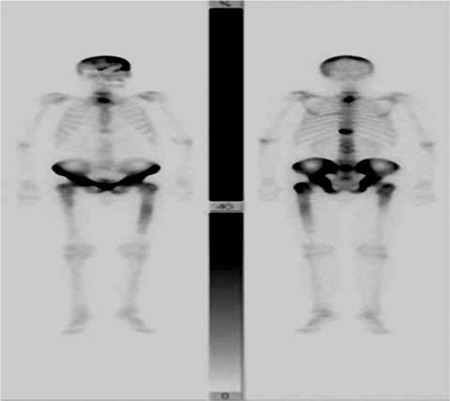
Dense and diffuse increased osteoblastic activity in the cranium; all pelvic and sacroiliac joints; the C7, T1-2, and T11 vertebrae of the vertebral column; and both proximal femurs on technetium-99m bone scintigraphy.
